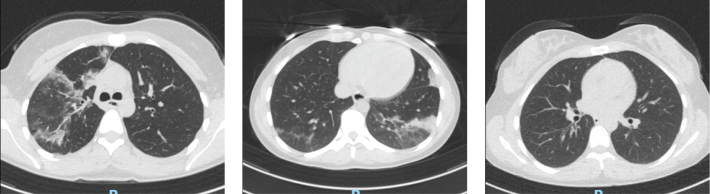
CT scans of Case 1 in (a) June, (b) August, and (c) October, demonstrating a pattern interpreted as organizing pneumonia that initially progressed and then regressed after antiviral treatment.
